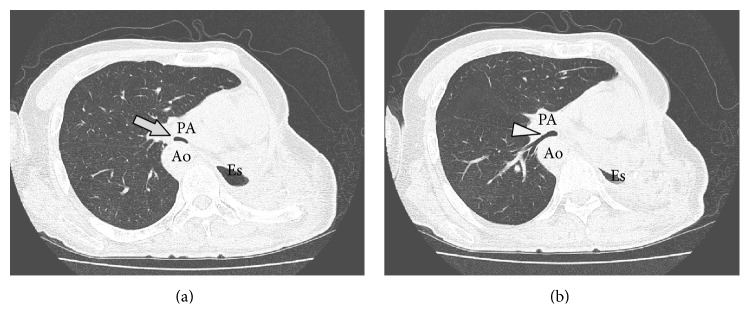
Computed tomography taken two months after the thoracoplasty. The bronchus intermedius ((a) arrow) and right lower bronchus ((b) arrowhead) are patent, and there is no relapse of postpneumonectomy syndrome. Ao, right descending aorta; PA, right pulmonary artery; Es, esophagus.
